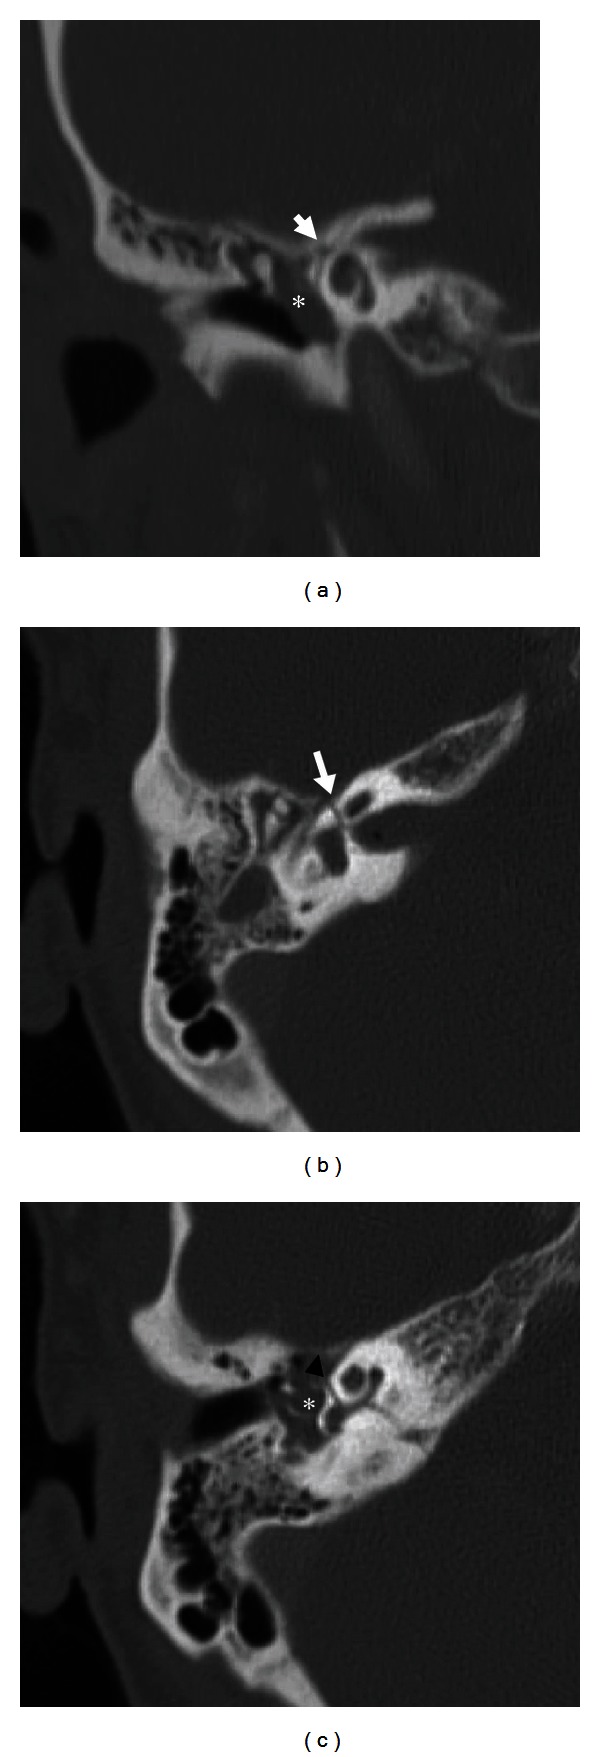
Transverse temporal bone fracture. Coronal (a) and axial (b) CT images demonstrate a transverse fracture through the labyrinthine segment of facial nerve canal (arrows). Axial CT image (c) shows the fracture involving the otic capsule and basal turn of the cochlea (arrowhead). Note blood products in the middle ear cavity (asterisks).
